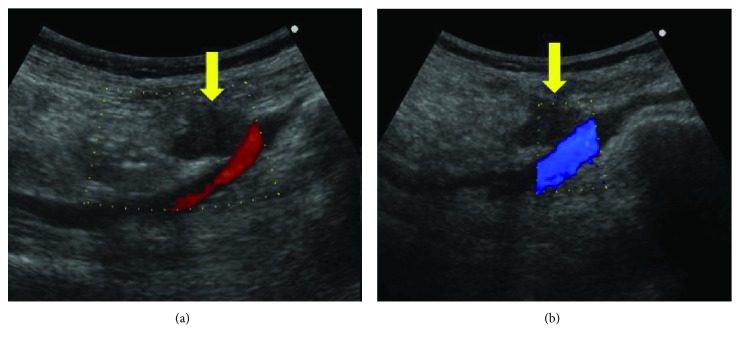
Ultrasonography showing a 27 × 16 mm, irregular, low-echoic tumor (arrow). The tumor is located around the external iliac artery (a) and the external iliac vein (b).
